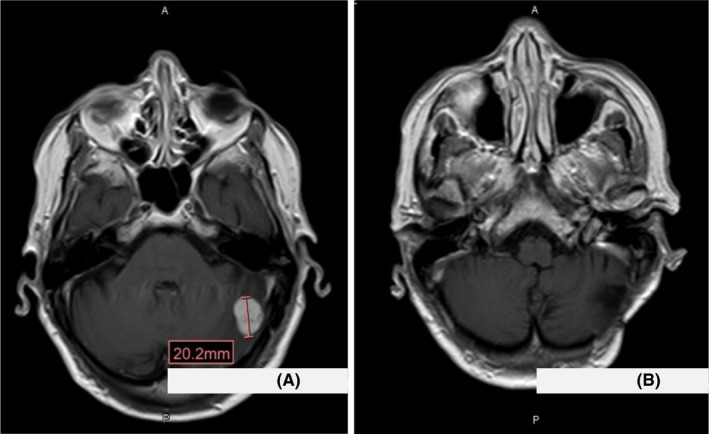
Cranial magnetic resonance images. (A) Shows the metastatic lesion in the left cerebellar hemisphere (January 2019). (B) Shows the absence of residual disease in the surgical bed during follow‐up (July 2019).
